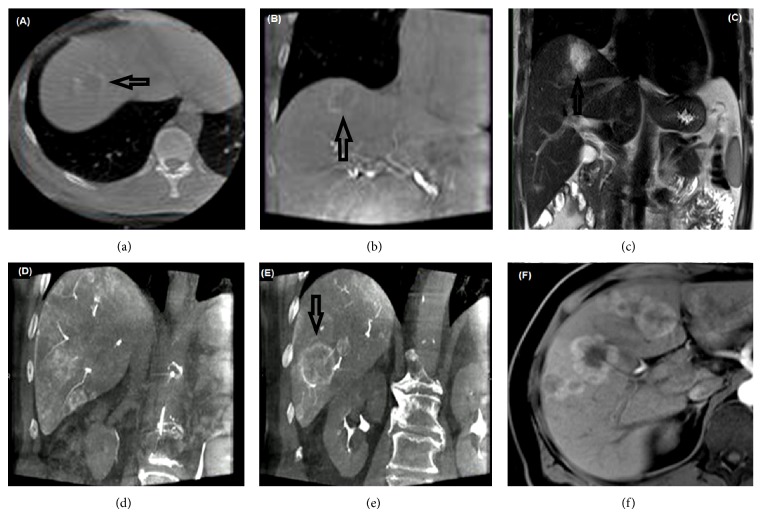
Frames (a) and (b) represented the images obtained from a 62-year-old patient during TACE therapy, generated using ultrafast cone-beam CT with imaging protocol 1. Pretreatment magnetic resonance cross-sectional images ((c) and (f)) show a clear view of embedded tumor in the hepatic parenchyma. Hepatic tumor detection was insufficient using imaging protocol 1 data (tumor indicated using black arrow) compared to imaging protocol 2 due to a reduction of contrast material in the tumors. Coronal reconstructed images ((d) and (e)) were acquired using imaging protocol 2 during a 60-year-old patient TACE examination. The images show excellent tumor(s), feeding vessels, and hepatic parenchymal visualization; furthermore, notice the strong contrast material enhancement of the tumors with little or no artifacts.
